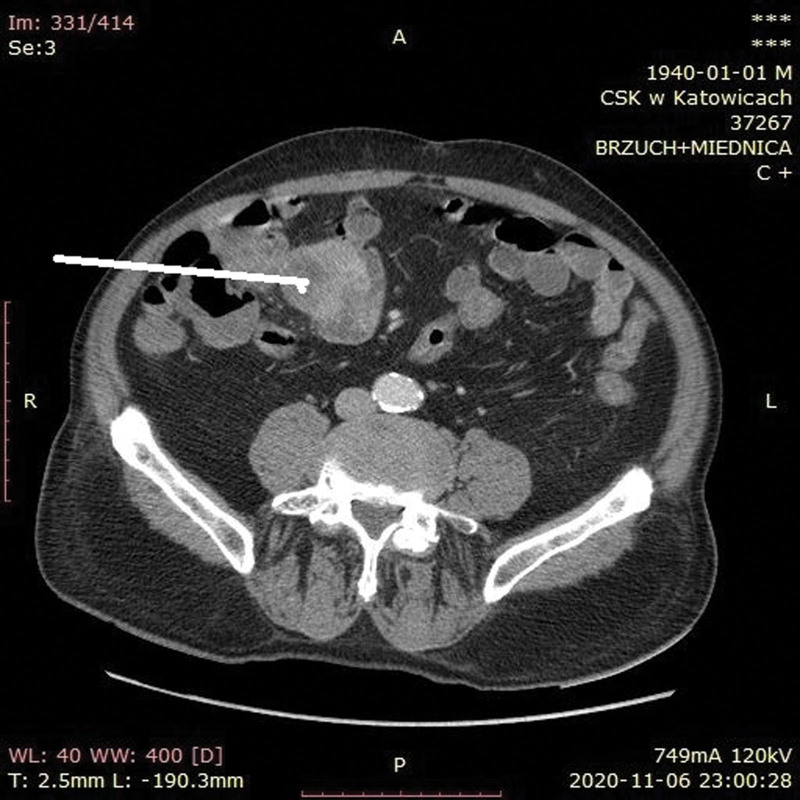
A multidetector contrast-enhanced CT of the abdominal and pelvic cavity showing concentric irregular thickening in the distal jejunum (white arrow).
